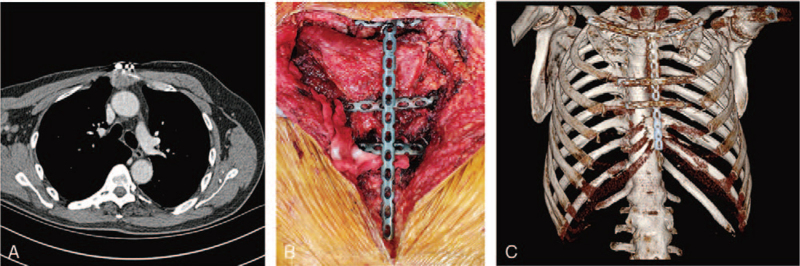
(A) Chest CT revealed tumor recurrence again. (B) The third operation of tumor resection and chest wall reconstruction. (C) CT review after the third operation.
